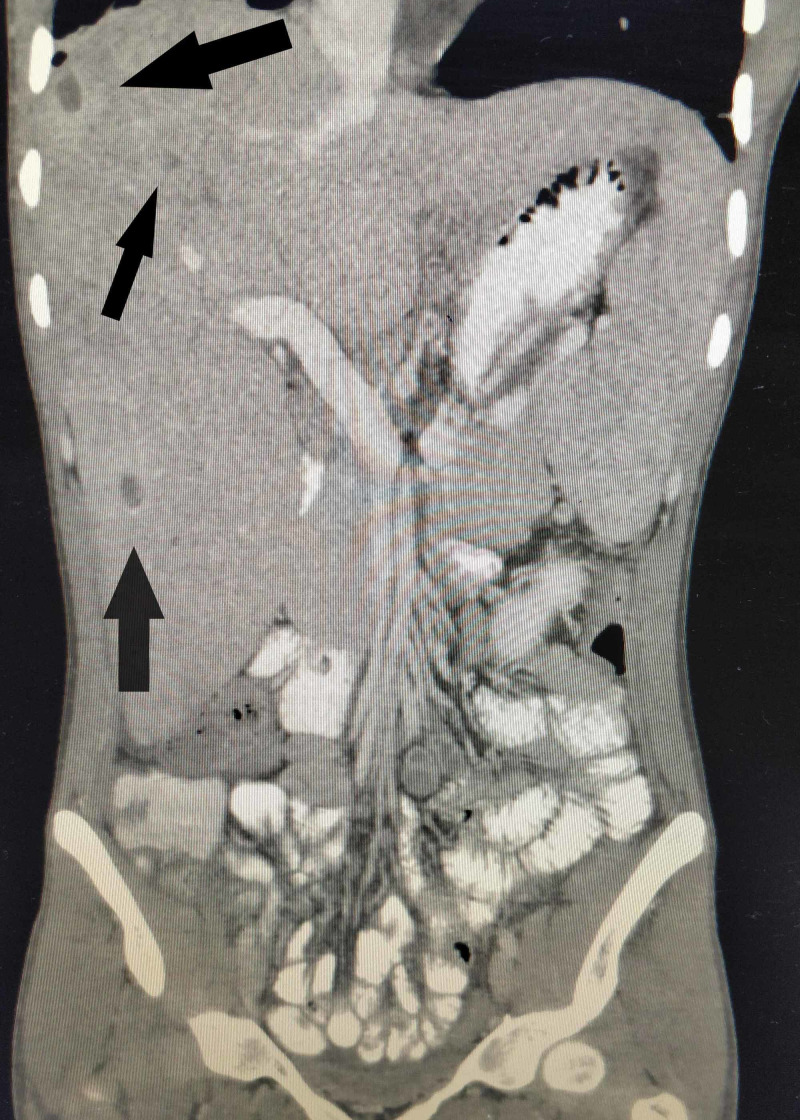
Coronal section of contrast-enhanced CT scan of the abdomen Microabscesses in the liver (black arrows) visible as small round, hypodense lesions with minimal peripheral enhancement.
